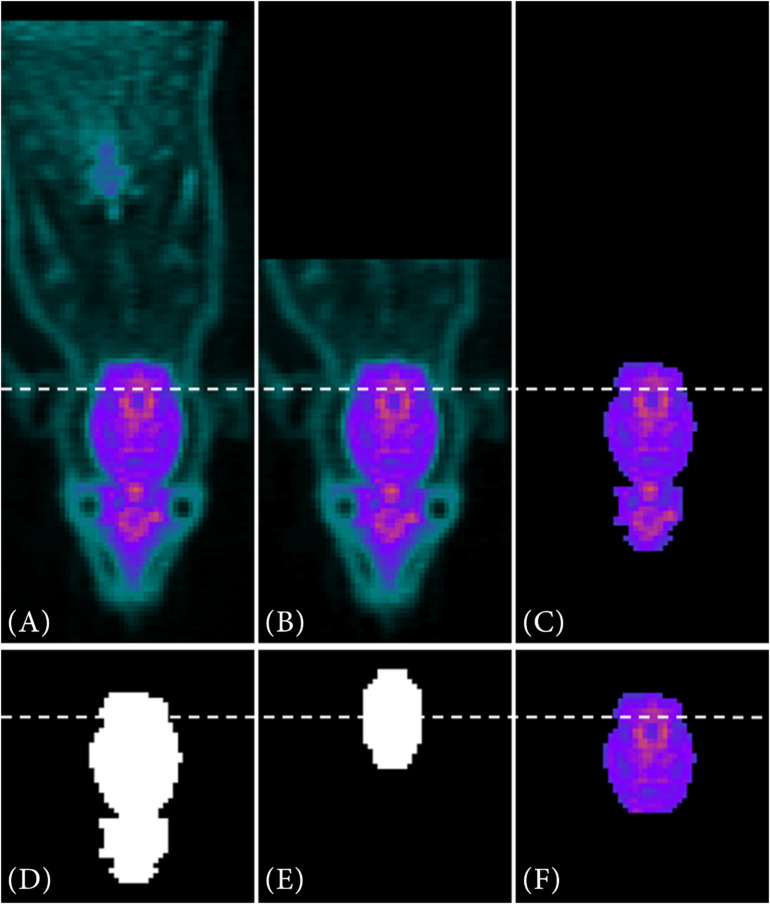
VOI segmentation steps for a metabolic radiotracer. In (A) the full PET image is depicted. After data reduction (B) and automatically threshold adaption using the Otsu method (C) a binary image is created out of the reduced data (D). Via contour matching with the resized MRI atlas set as a binary image (E), the VOI is segmented (F).
